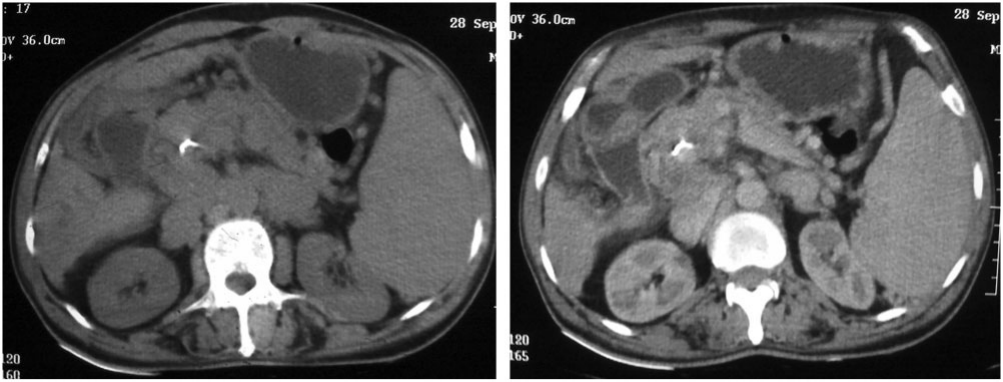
Plain and contrast CT images showing heterogeneously enhancing lesion in the pancreatic head region and calcification of portal vein.
